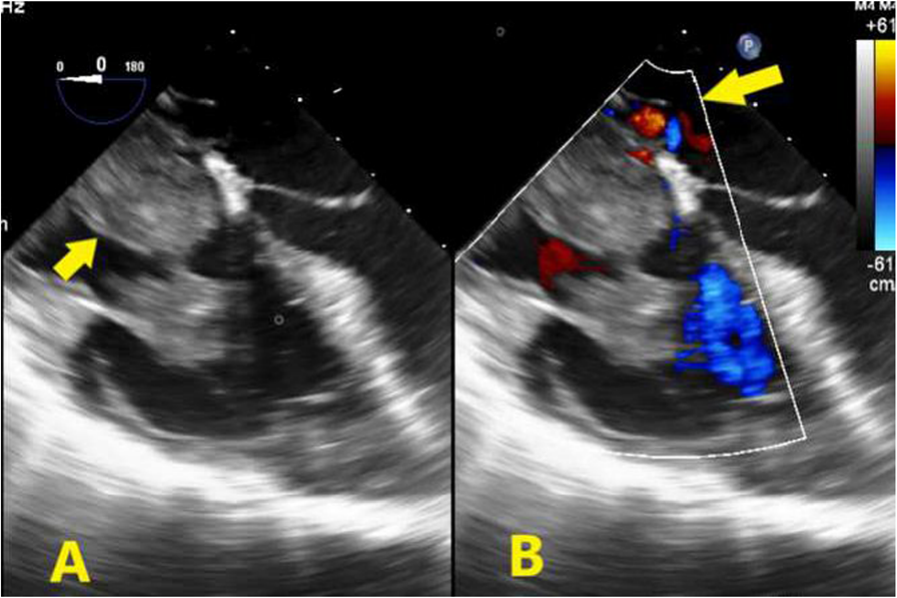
a Trans-esophageal two-dimensional echocardiography, mid-esophageal apical 4-chamber view showing a huge right atrial mass filling the whole cavity of the right atrium oscillating in and out of the right ventricle through the tricuspid valve. b Color Doppler showing patent foramen ovale
